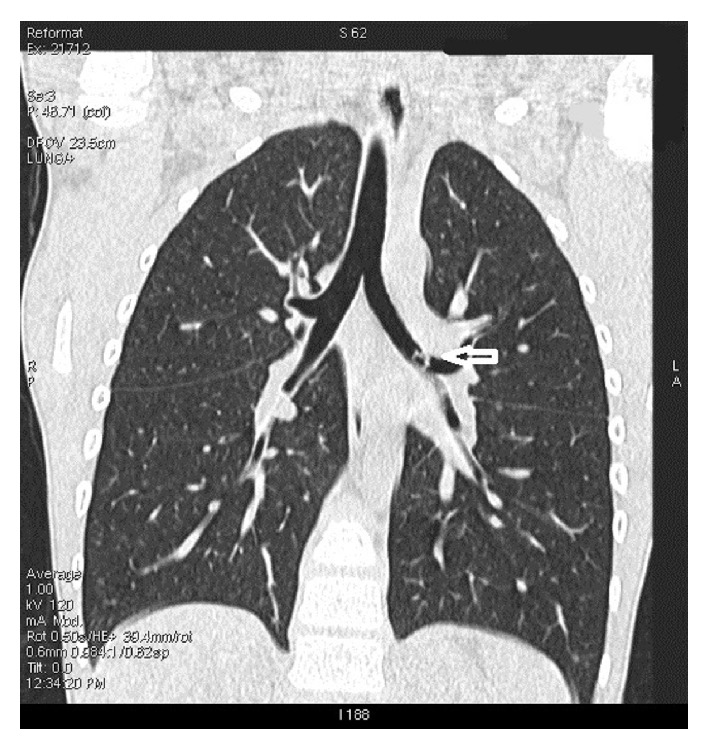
A low-dose CT thorax, at coronal plane, showing an intraluminal foreign body with an inverted U appearance (arrow) lodged at the distal left main bronchus measuring 5.4 × 6.8 × 7 mm.
